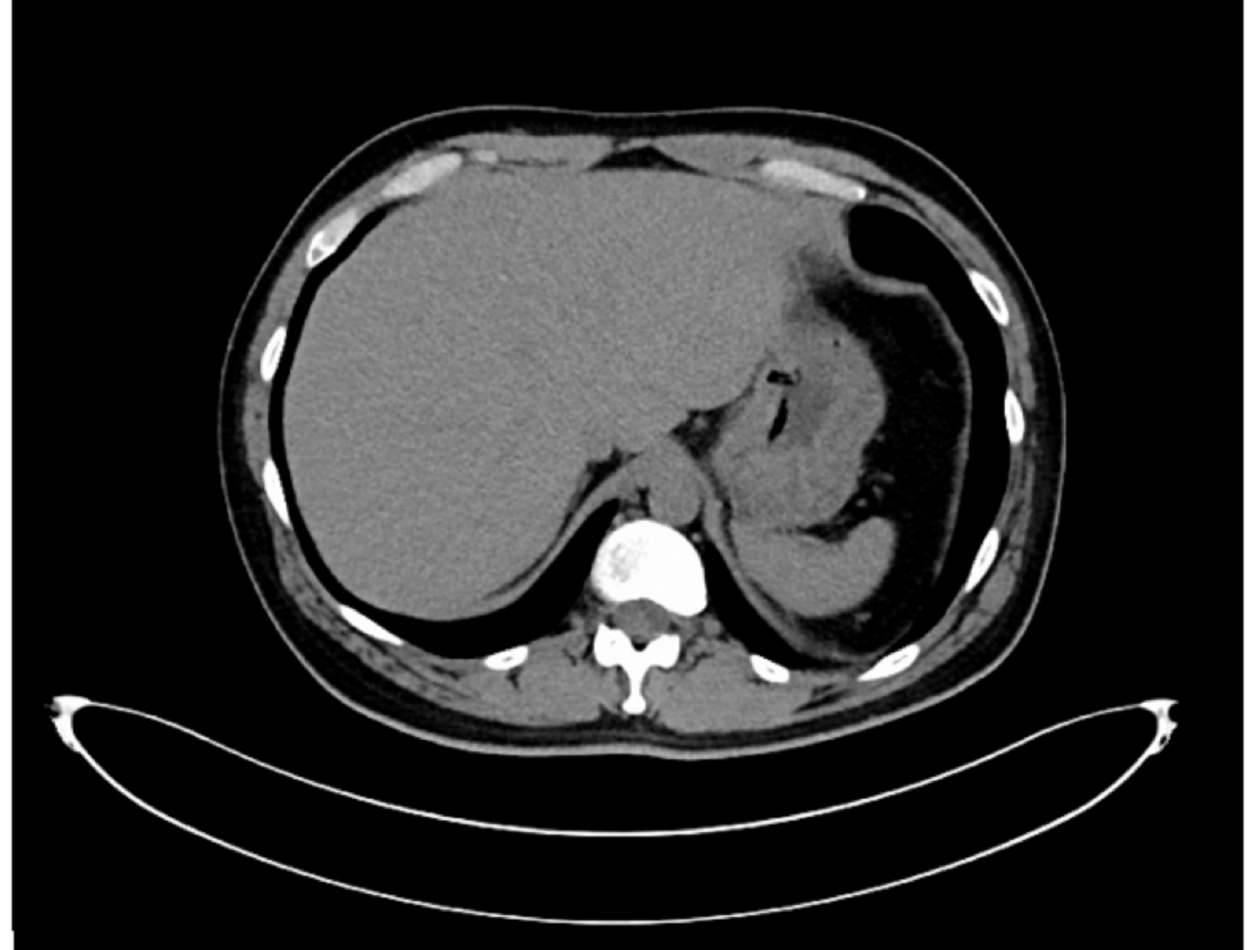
CT abdomen done in July 2019. Depicting no liver mass or abscess seen.
